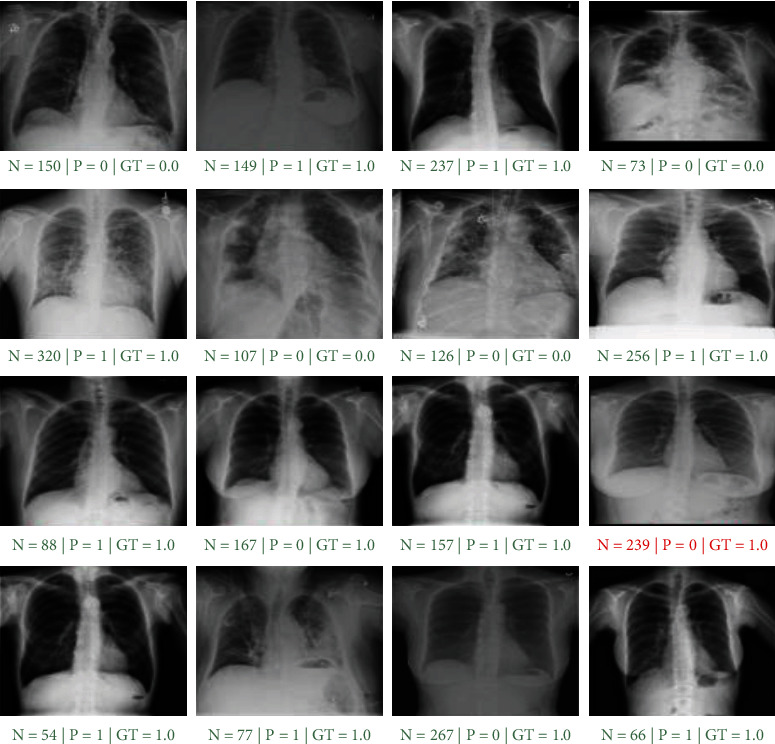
The first phase performance evaluation results on 16 X-ray random samples. *N*, *P*, and GT are image index, model prediction, ground-truth label, respectively. The green caption indicates accurate prediction, while red shows the inaccurate prediction.
